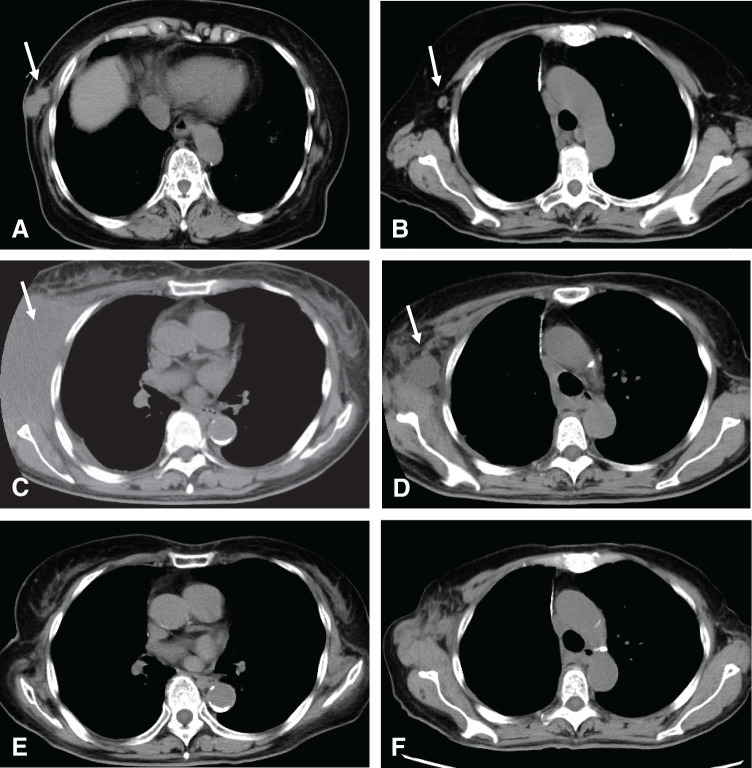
Sequential CT images over time. The left row shows chest wall recurrence (arrows), and the right row shows axillary lymph nodes (arrows). (A), (B) Findings at recurrence, 4 months postoperatively. (C), (D) At the time of regrowth of the chest wall tumor and axillary lymph nodes, 16 months after initiation of second line systemic therapy. (E), (F) At the time of response after 6 months of third line systemic therapy.
